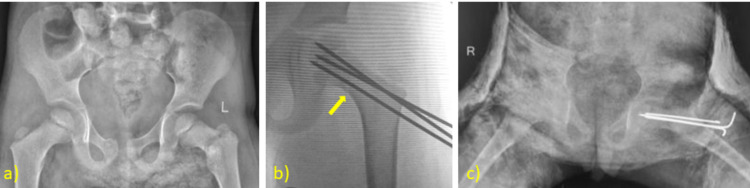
Case Example: Closed Reduction and K-Wire Fixation a) A five-year-old patient with Delbet type II fracture presented 115 hours after the injury and was taken to the operating room for fixation. Closed reduction was possible and stable fixation was achieved. b) The intraoperative c-arm image showing reduction and fixation. A yellow arrow is highlighting the fracture line. A hip spica cast was applied postoperatively. c) AP radiographs showing adequate reduction. AP: anteroposterior
